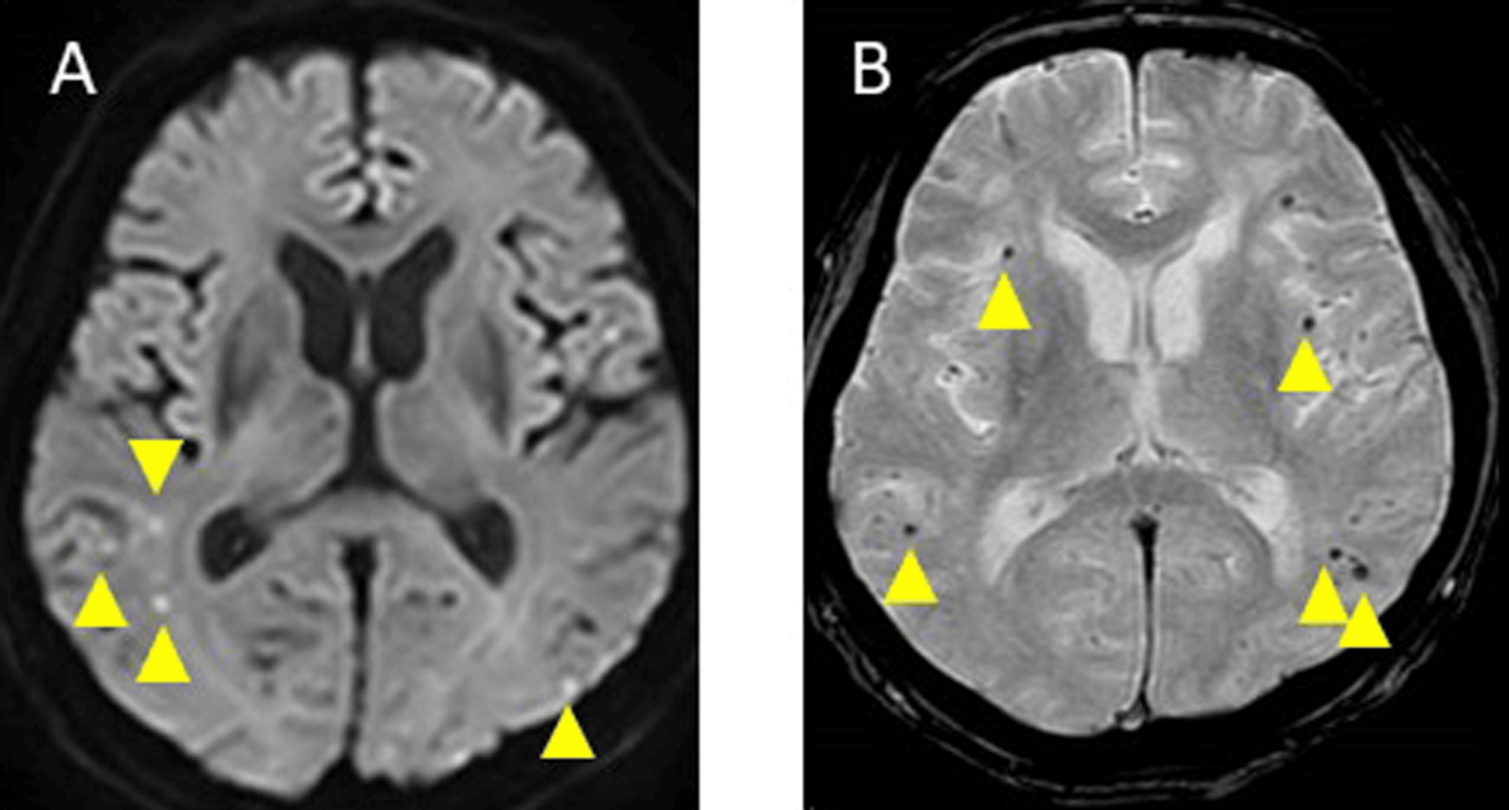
Brain magnetic resonance imaging diffusion-weighted imaging (DWI), and T2*, on day 73 A: MRI-DWI image showed an increase in small high signals in both occipital and parietal lobes (yellow triangle). B: T2* imaging demonstrated an increase in microbleeds in both frontal parietal lobes (yellow triangle).
